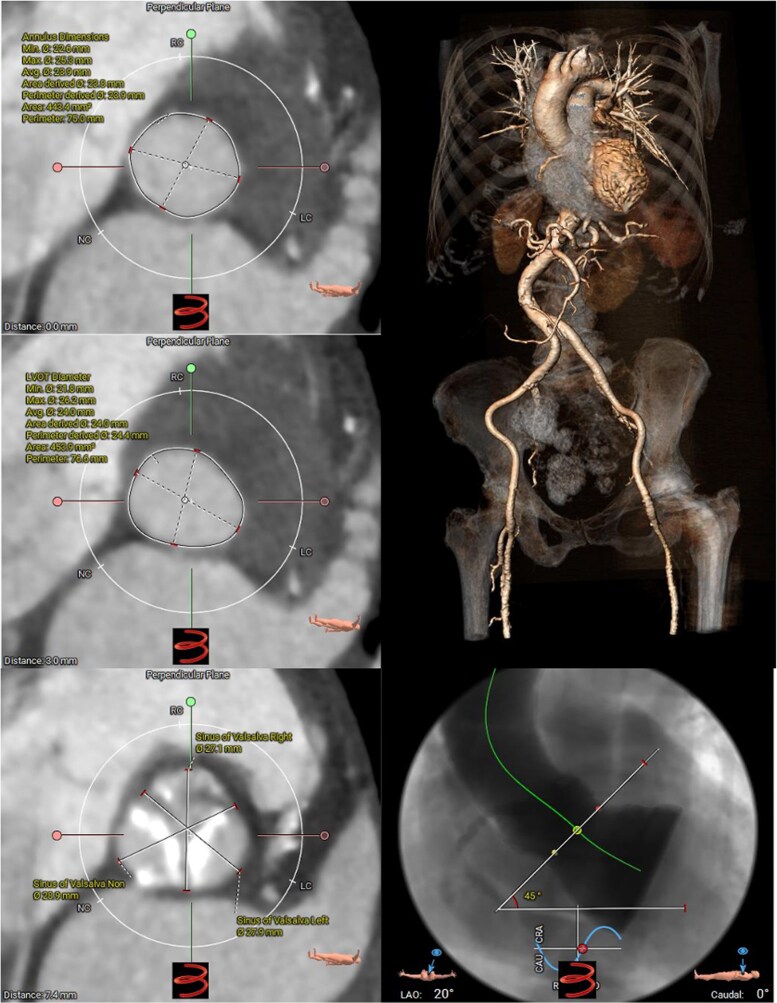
Case 1: CT TAVI for Case 1. Case planning highlighted significant tortuosity of the iliac vessels and aorta. A 23 mm Sapien was chosen based on the sizing in the upper end of the range, very severe leaflet calcification, and no LVOT or annular calcification.
